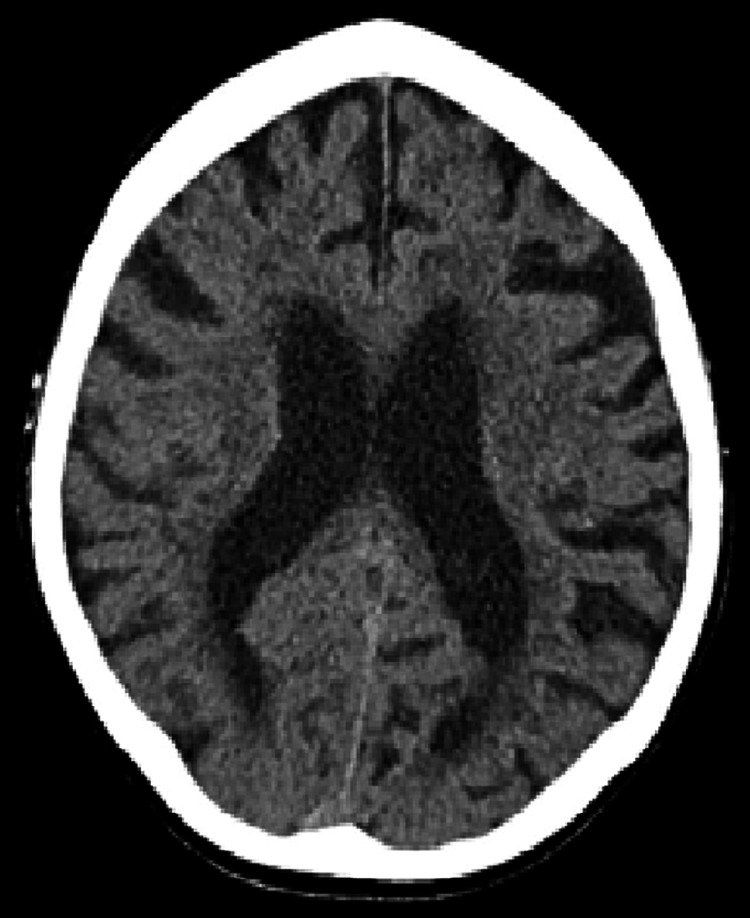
Axial CT brain on day one of admission demonstrating moderate periventricular hypodensity in keeping with chronic small vessel ischemia and age-appropriate involutional changes aside from prominence of sulci within the left hemisphere, suggestive of prior infarction.
